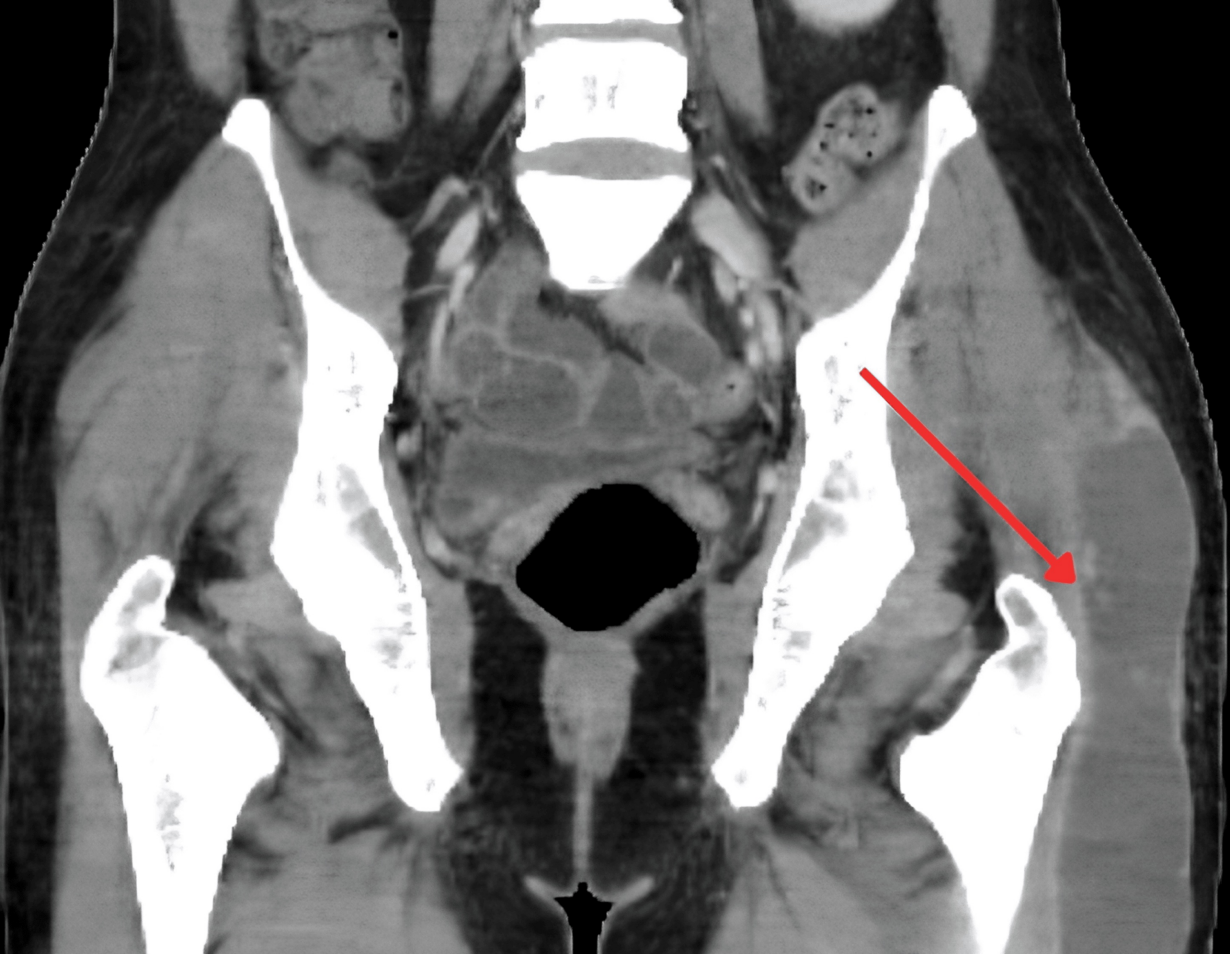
CT showing large thick-walled low-density and peripherally enhancing collection overlying the left greater trochanter, likely tuberculous bursitis. The lesion supports systemic dissemination of TB. TB: Tuberculosis
